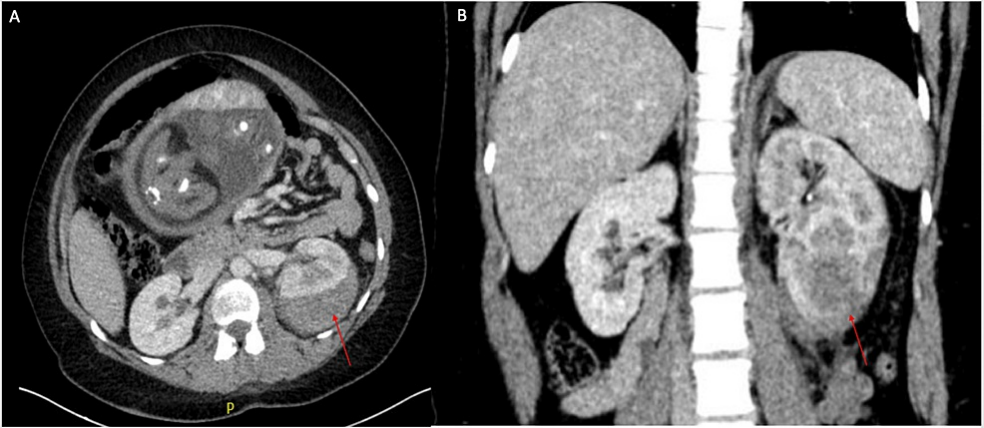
Low-dose CT showing axial (A) and coronal (B) views of the large subcapsular hematoma (red arrow)
